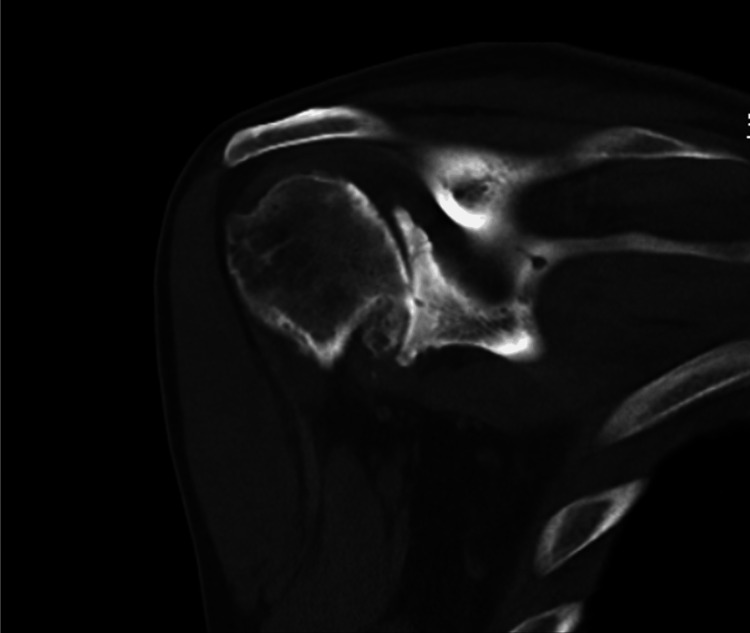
Preoperative CT of the right shoulder CT: computed tomography The image depicts a coronal view of the right shoulder
